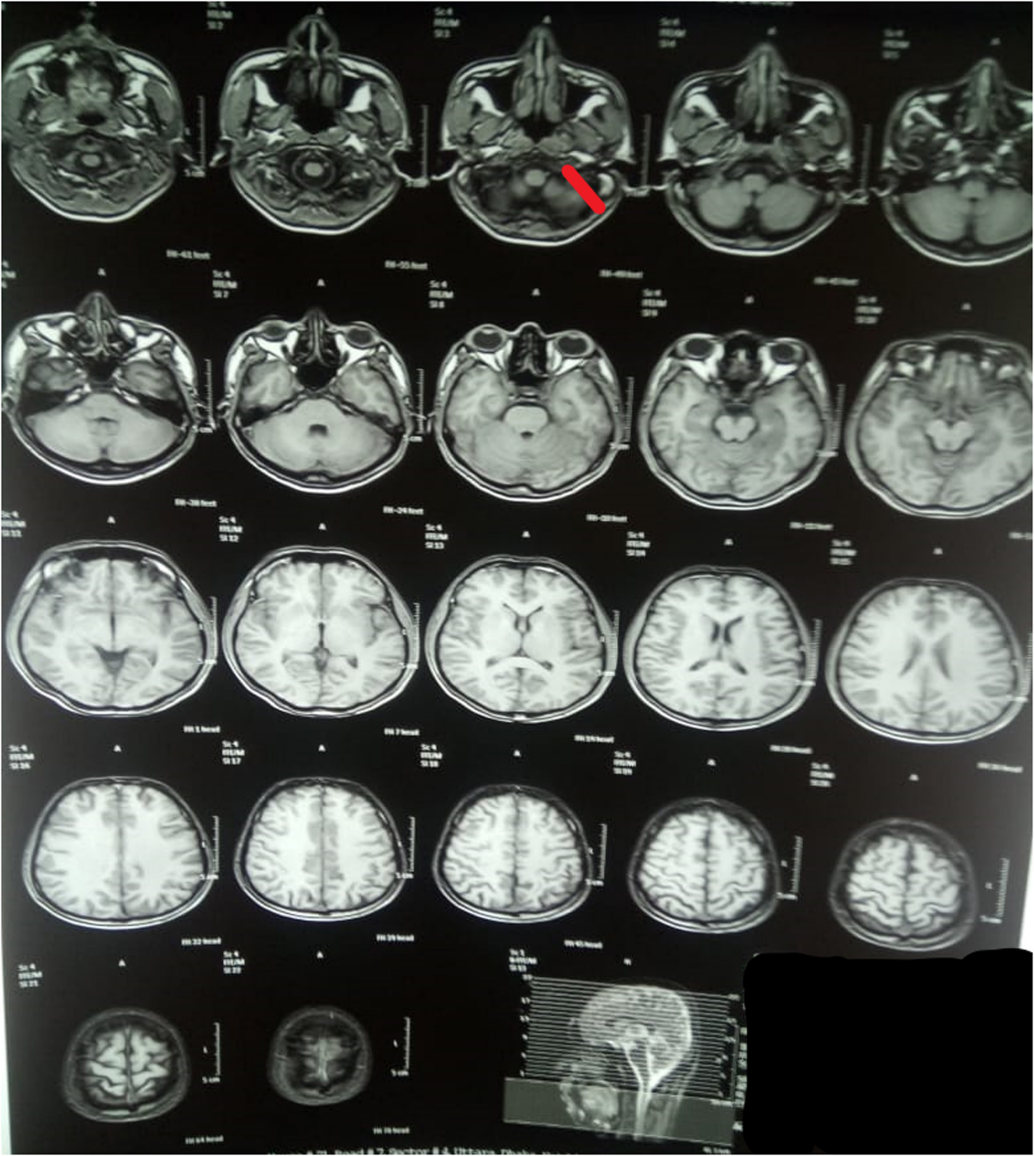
Magnetic resonance imaging (MRI) scan demonstrating absence of enhancement in the left internal jugular vein (IJV).
